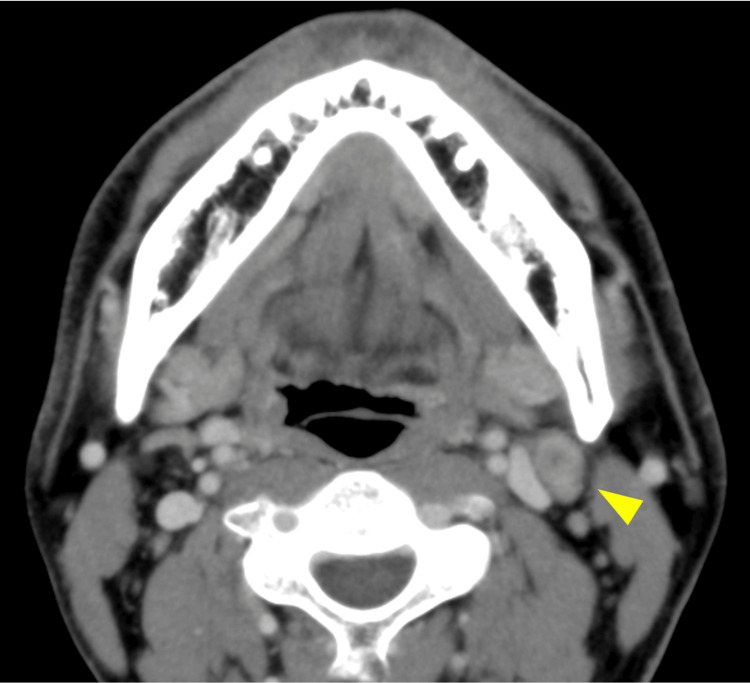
Imaging findings of late lymph node metastasis Contrast-enhanced computed tomography (CT) showing enlarged lymph node suspicious for central necrosis in level Ⅱ lesion (arrow head).
